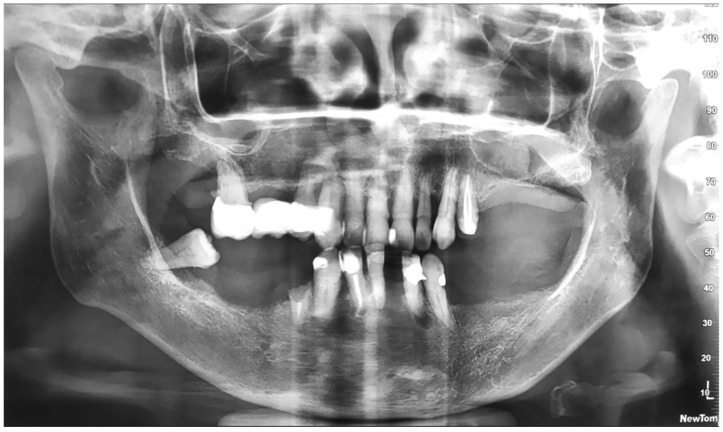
Panoramic radiograph (January 2020).
